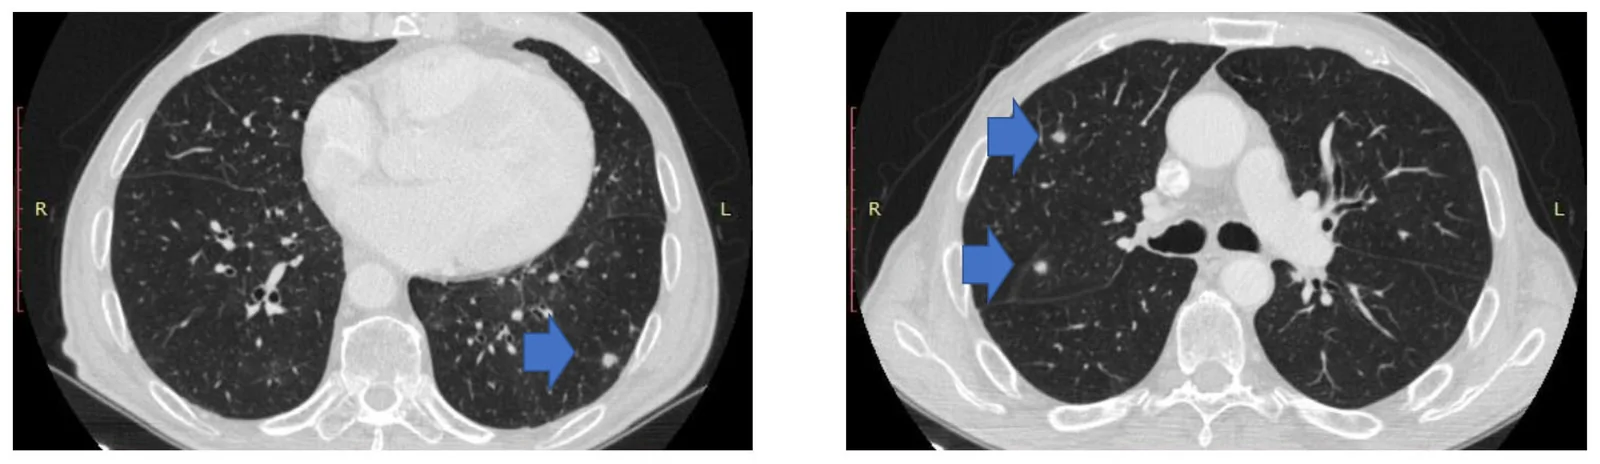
Representative baseline chest computed tomography images of Patient 4 before initiation of trastuzumab deruxtecan. Arrows indicate pulmonary metastatic nodules.
